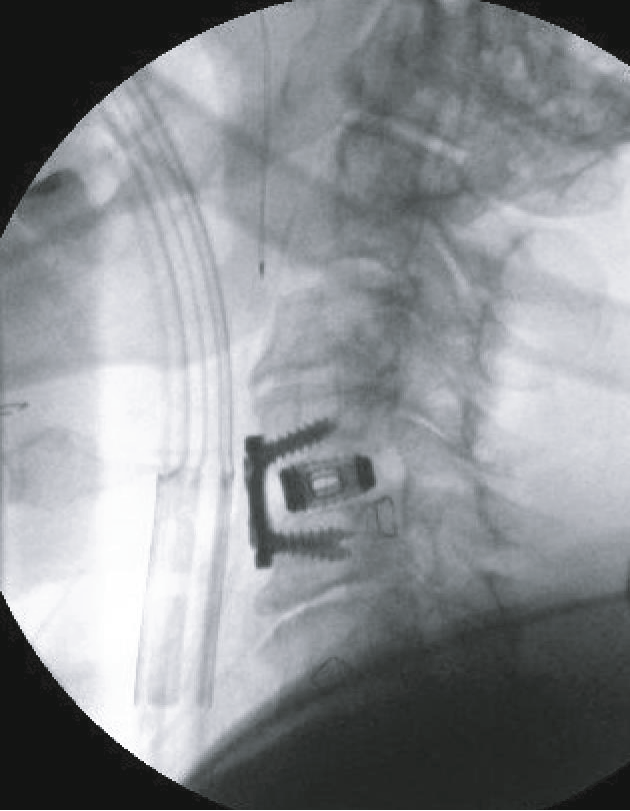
Lateral intraoperative radiograph of the cervical spine, status post-C4 and C5 anterior cervical discectomy and fusion.
